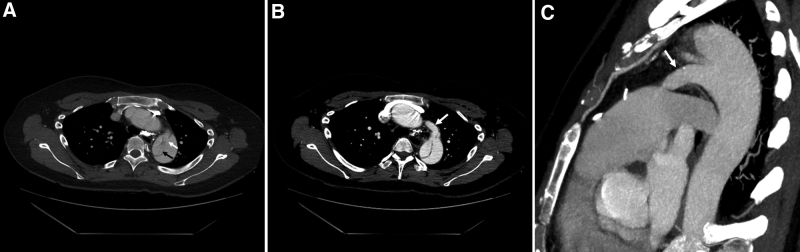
(A) CT revealing the dissection flap (black arrow) and entry tear site (white arrow) of the descending thoracic aorta. (B) Axial CT revealing PDA (arrow connected to the proximal descending thoracic aorta. (C) Sagittal CT revealing PDA (arrow), which has a narrow connection to the pulmonary trunk and a wide connection to the aorta. CT = computed tomography, PDA = patent ductus arteriosus.
